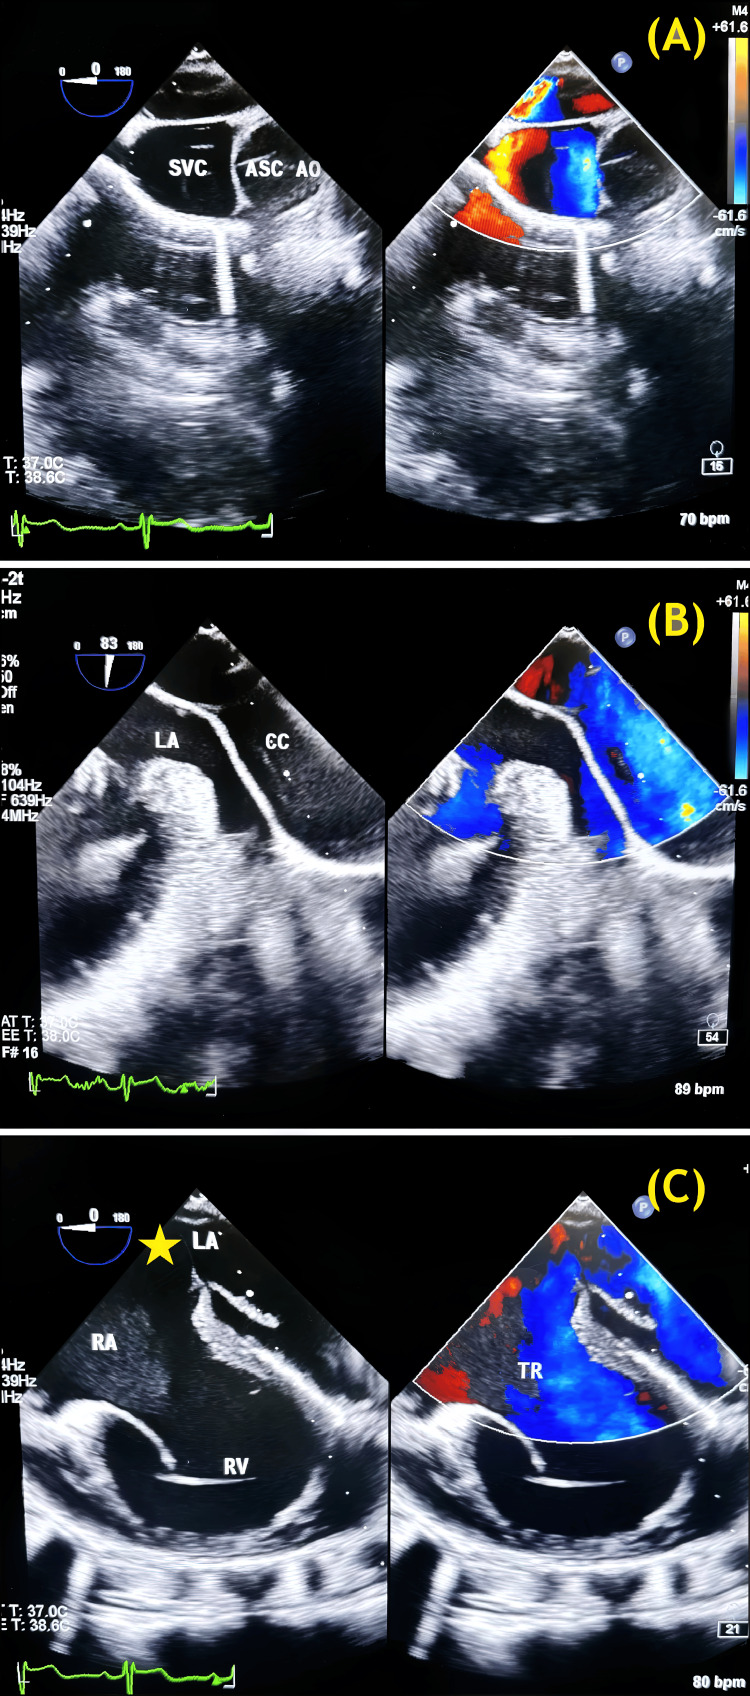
Preoperative TEE images showing (A) a dilated SVC; (B) separation between the CC and LA; and (C) the LA and RA, with a large unrestrictive ASD (starred) between them. TR is also seen. SVC: Superior vena cava; AO: Ascending aorta; CC: Common chamber; LA: Left atrium; RA: Right atrium; RV: Right ventricle; TR: Tricuspid regurgitation; ASD: Atrial septal defect; TEE: Transesophageal echocardiography. Image credit: Dr. Sambhunath Das
